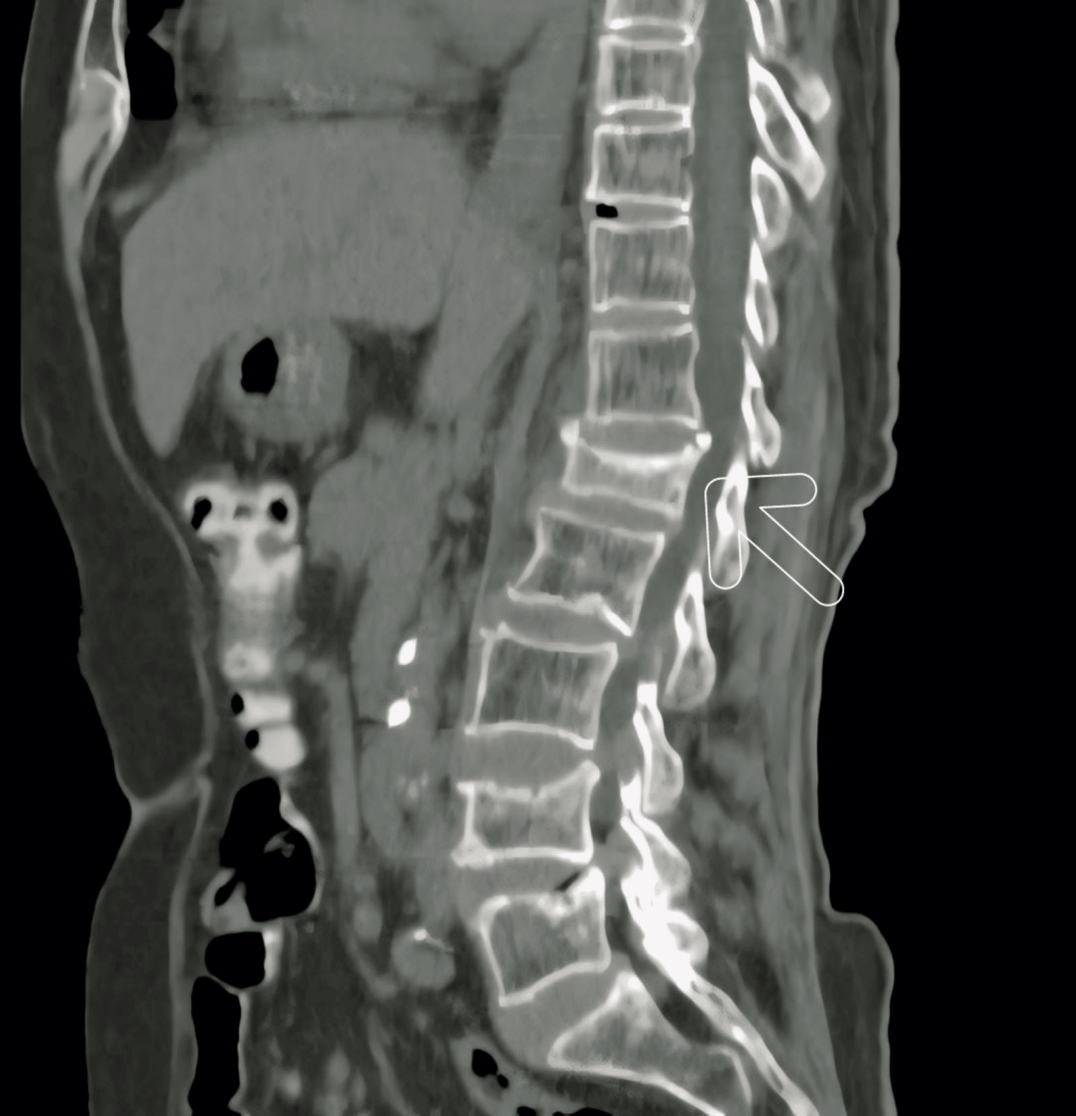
Computed tomography (CT) of the lumbar spine The arrow indicates the central wedge compression fracture of the L1 vertebral body and generalized osteopenia.
